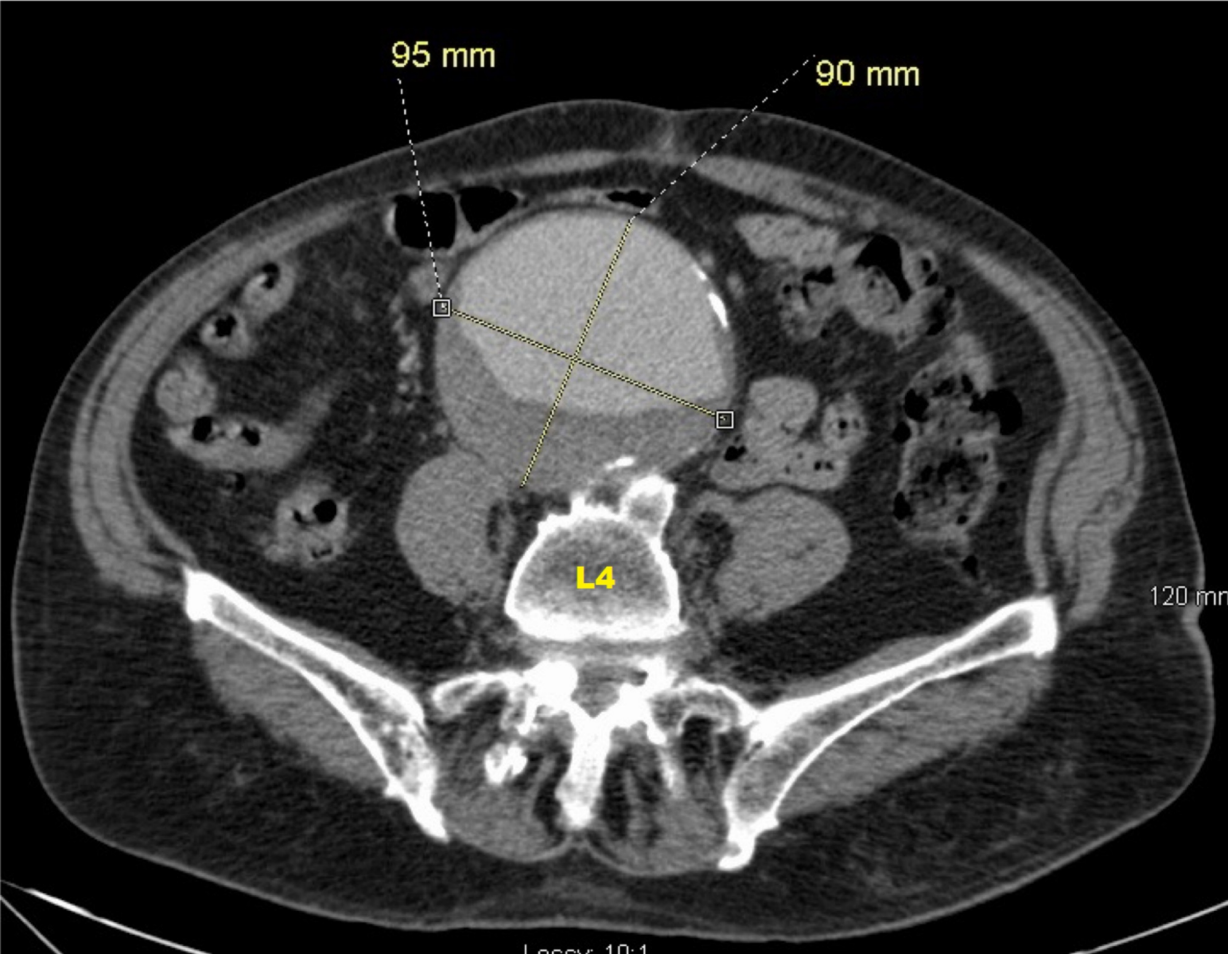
Contrast-enhanced axial CT of the abdomen and pelvis (level of L4) image showing 9.5 x 9.0 cm infrarenal AAA with peripheral mural thrombus AAA: abdominal aortic aneurysm; CT: computed tomography
